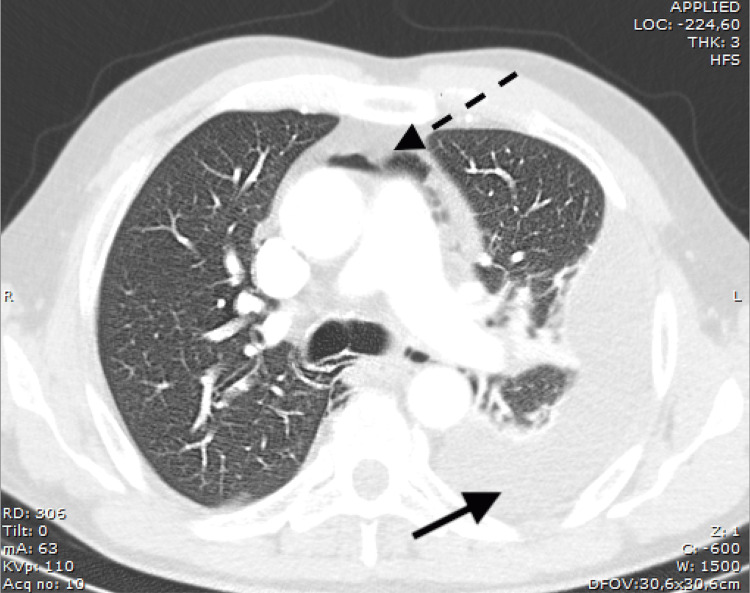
Axial CT acquisitions revealing a pneumomediastinum (dashed arrow) and a left pulmonary collection (bold arrow).
